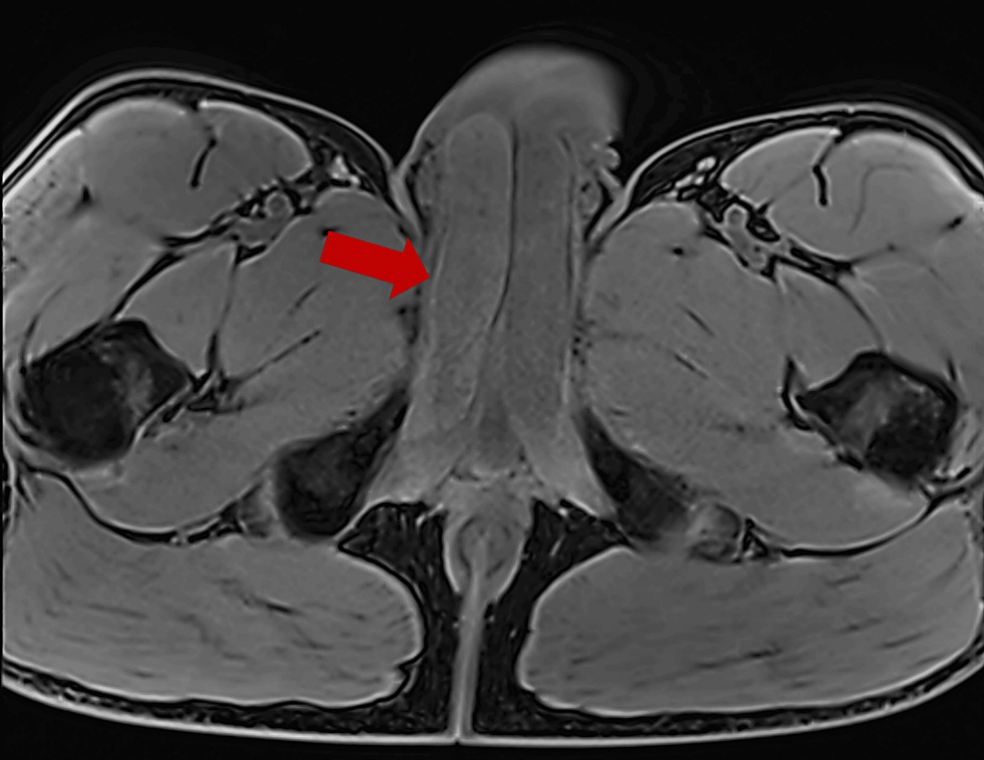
Transverse fat-saturated T1-weighted MRI. Enlarged appearance of the proximal portion of the right corpus cavernosum compared to the left with no definite T1 signal abnormality. A red arrow points at the right corpus cavernosum.
